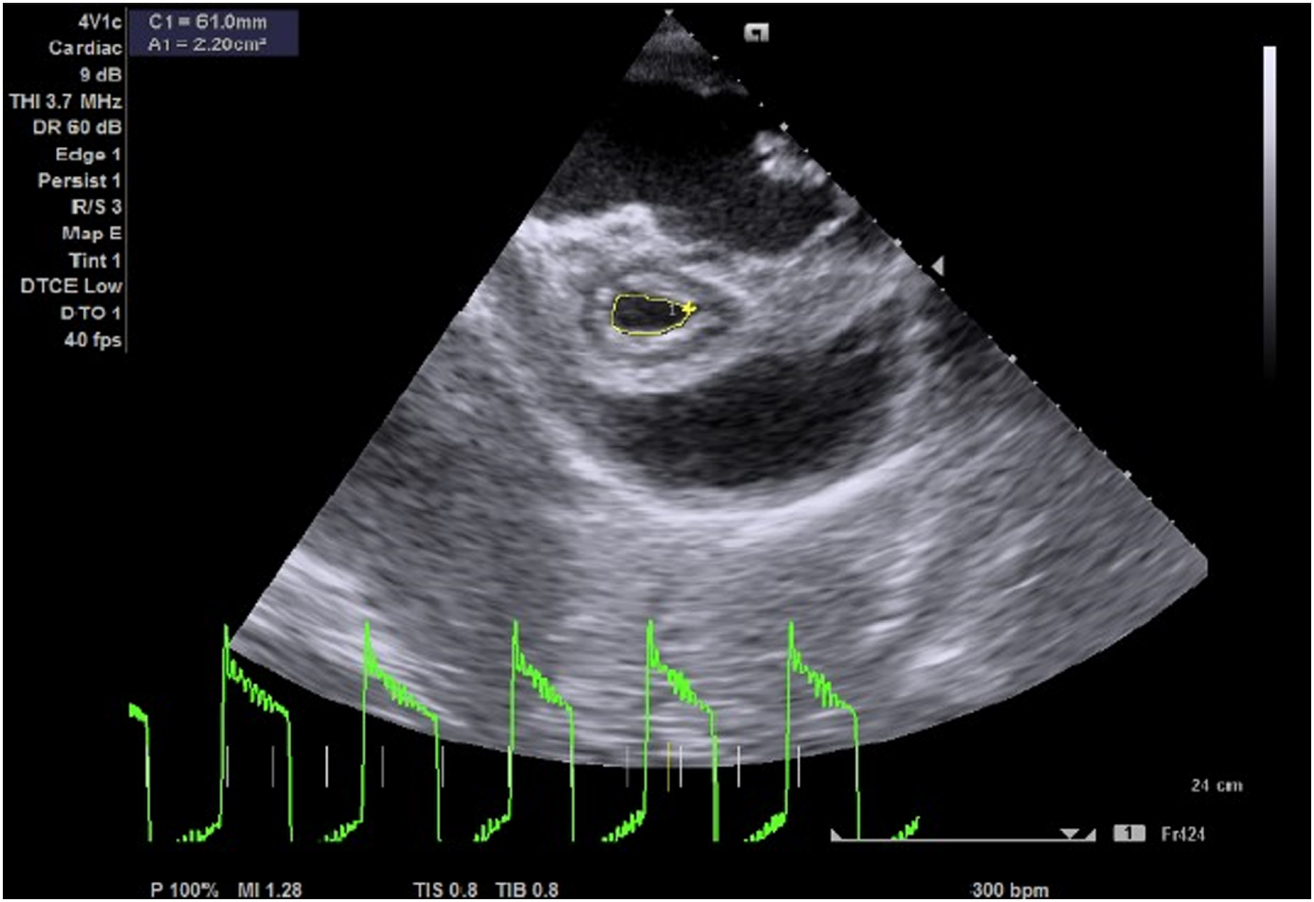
Parasternal short axis view (PSAX) echocardiogram showing mitral valve planimetry with mitral valve area of 2.2 cm^2^.
